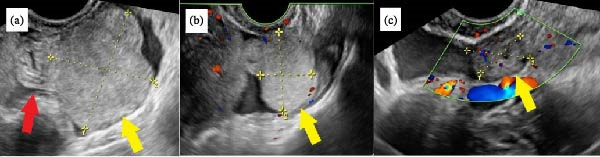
Diagnostic imaging findings. (a) Transvaginal ultrasound image showing a mass (yellow arrow) located in the subserosal area of the fallopian tube (red arrow); (b) color Doppler image showing no blood flow to the mass (yellow arrow); (c) color Doppler image showing reserved blood flow to the fallopian tube (yellow arrow).
